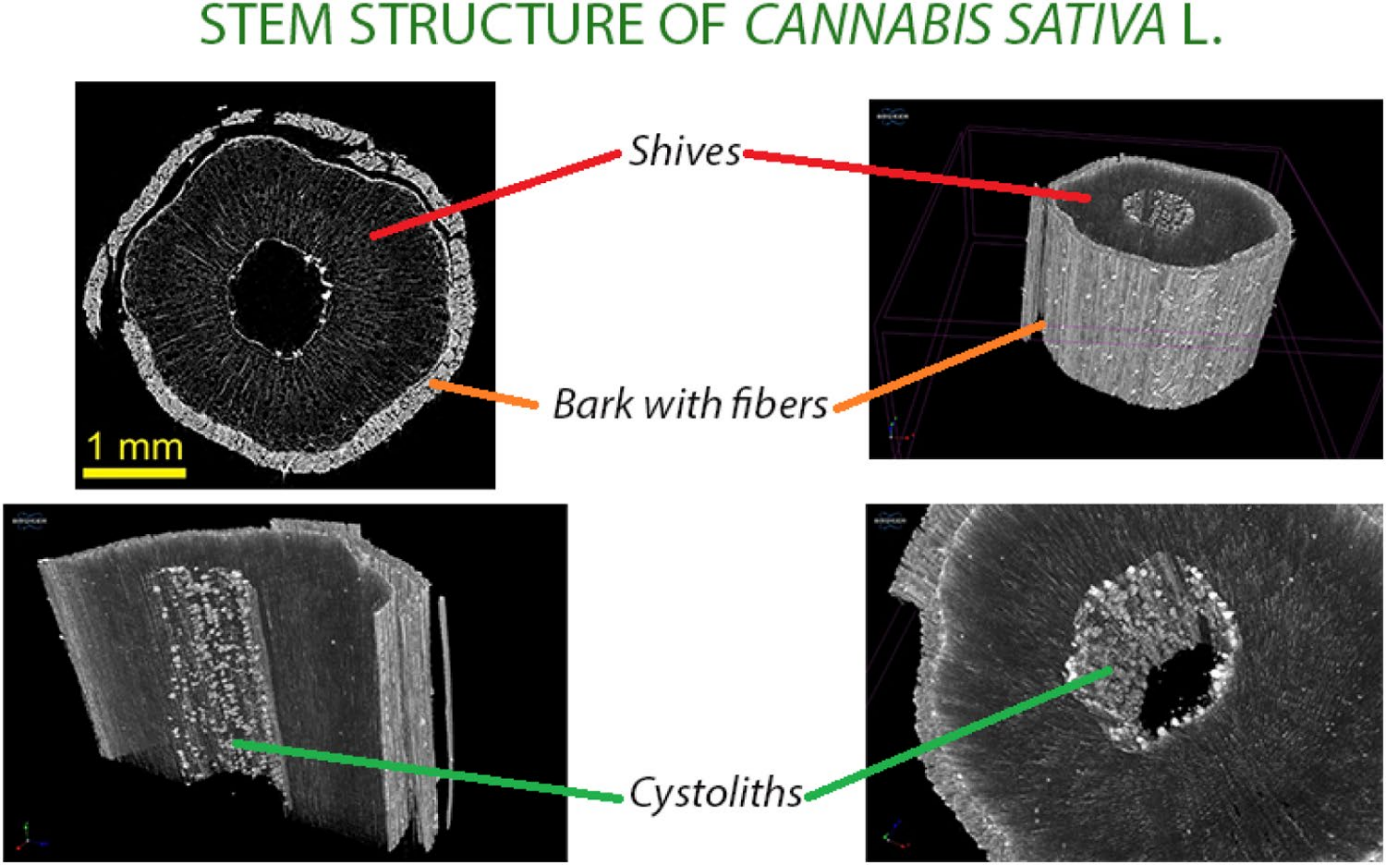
The 3D structural survey of the characteristics of stem of *Cannabis sativa* L. cultivar Uso 31 for an evaluation of the hemp/fiber ratio. By kind permission of Dr. Giacomo Mele responsible for the CNR-Isafom X-ray Microtomography Laboratory
